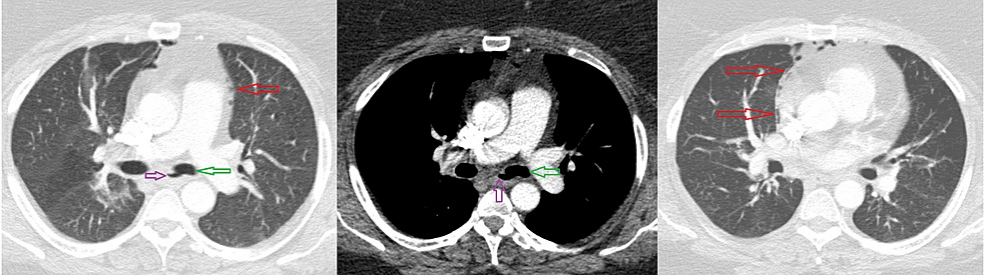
Computed tomography showing pneumomediastinum (red arrows) which may be secondary to a connection between the esophagus (purple arrows) and left main stem bronchus (green arrows).
